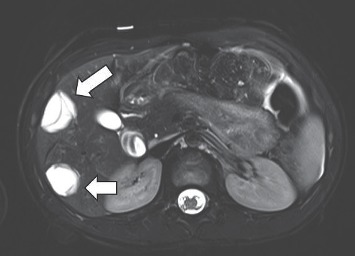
MRI scan of the patient no.3: multiple cysts up to 2.7 cm in S8/5, S7/6, S7, and S2/4 of the liver.
